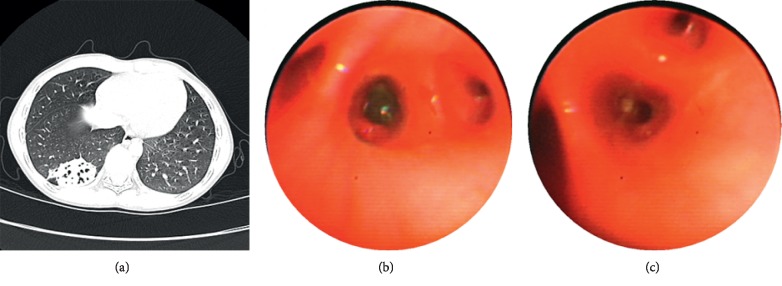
The images of CT and bronchoscopy of case 5: (a) shows bronchiectasis in posterior basal segment of the right lower lobe; (b) shows a spherical foreign body in the posterior basal segment of the right lower lobe (c) shows the picture after removal of foreign body.
